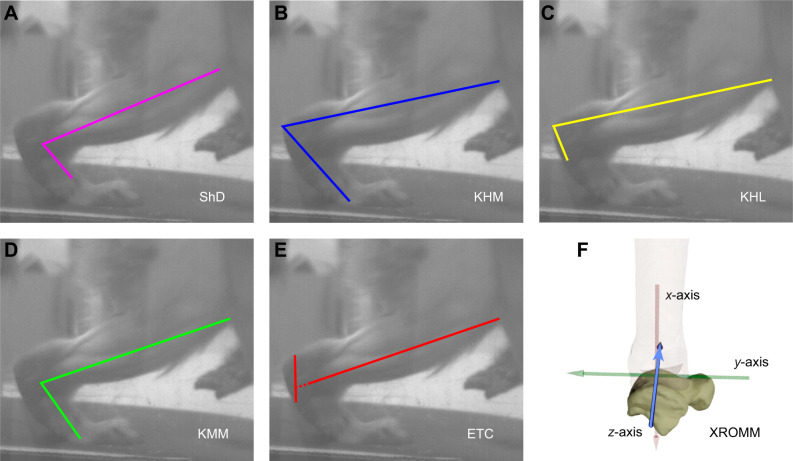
Six methods of measuring ankle dorsiflexion. (A) Shank to dorsum of the foot (ShD); (B) knee to heel to fifth metatarsal head (KHM); (C) knee to heel to lateral margin of the foot proximal to midfoot bend (KHL); (D) knee to medial malleolus to fifth metatarsal head (KMM); (E) angle between a line between the knee and the medial malleolus and a line between the superior posterior calcaneus and the intersection of the midfoot and hindfoot planes (ETC); and (F) X-ray reconstruction of moving morphology (XROMM) joint coordinate system. The *z*-axis (blue) is dorsiflexion.
